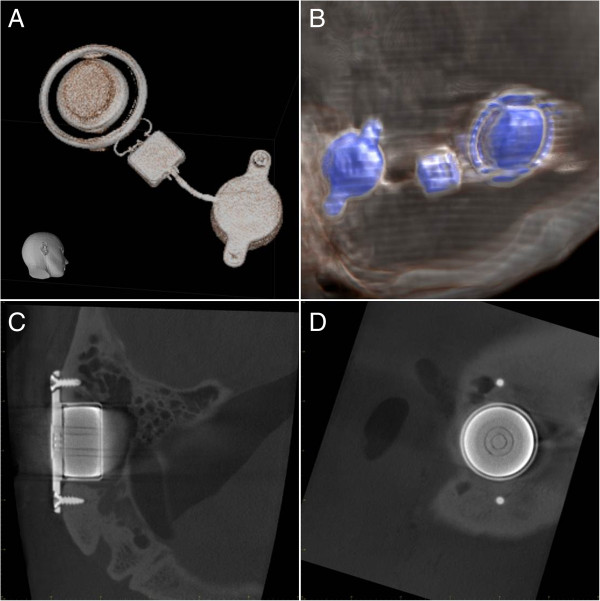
Visualisation of the implant. Three-dimensional reconstruction of the implant from the raw data of the CBCT (A) and CT (B). Imaging of the implant details including the inner structure and fixing screws in CBCT (C and D).
